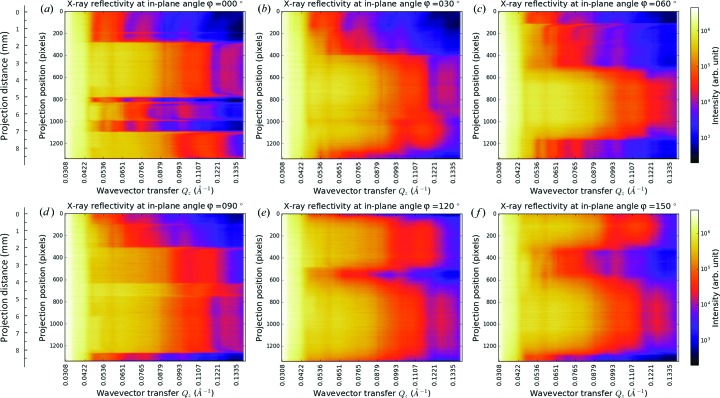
Selected X-ray reflectograms of the sample plotted as a function of wavevector transfer *Q_z_* at specific in-plane angles: (*a*) φ = 0°, (*b*) φ = 30°, (*c*) φ = 60°, (*d*) φ = 90°, (*e*) φ = 120° and (*f*) φ = 150°, where the data are plotted on the same logarithmic colour scale. The scanning step for the measurement is Δ*Q_z_* = 0.00114 Å^−1^.
